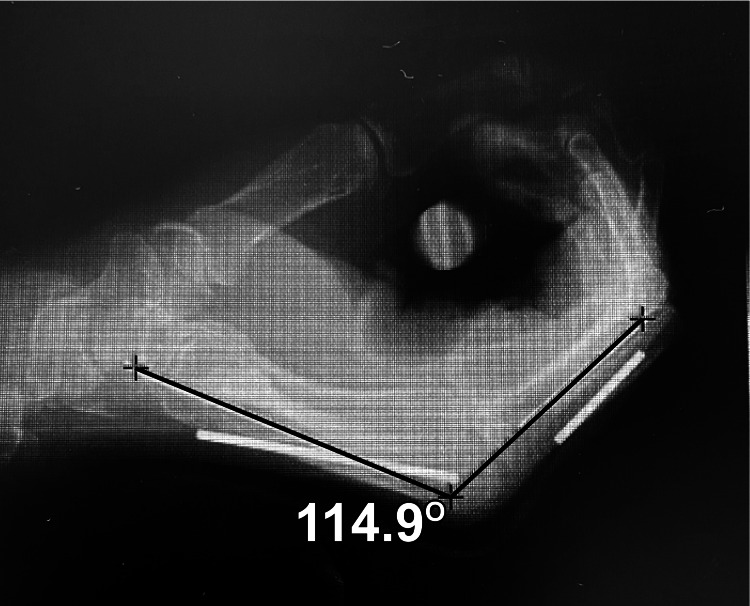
Measurement methods for the maximum range of motion at the index and little fingers Using a copper wire attached just above the basal and metacarpal bones of the index and little fingers, flexion and extension exercises were performed twice for five seconds. Using an X-ray fluoroscopy system, the maximum range of motion in flexion and extension of the index and little fingers during the exercise was measured.
